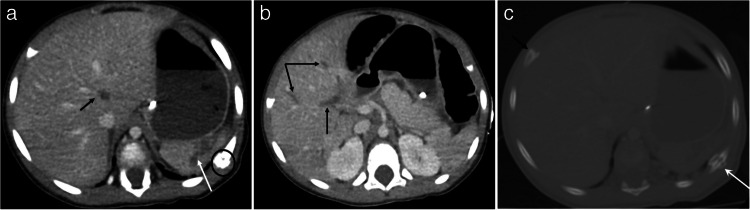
2-year-old male found unresponsive with chest wall bruising and elevated liver enzymes. Findings elicited concern for physical abuse. CT of the abdomen and pelvis in portal venous phase was obtained. a Axial image shows splenic (*white arrow*) and liver (*black arrow*) lacerations each consistent with grade II injuries and a healing rib fracture (*black open circle*). b Axial image of the same study at a slightly more caudal level shows multiple branching liver lacerations (*black arrows*) consistent with a grade II liver injury. c Axial CT image in bone algorithm at the level of the splenic laceration better delineates the healing left rib fracture (*white arrow*) and an anterior right rib fracture (*black arrow*)
